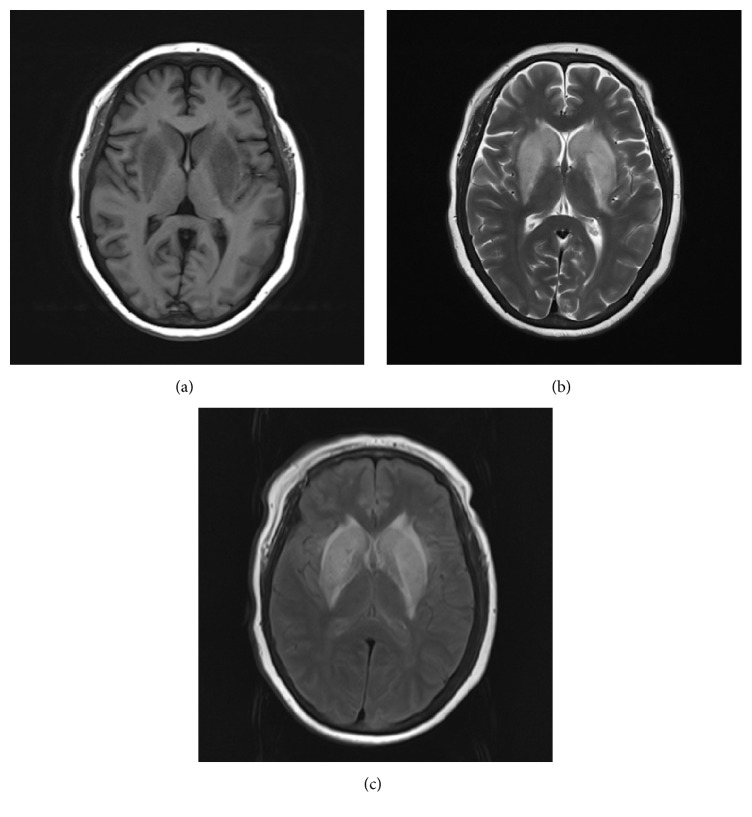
(a, b) The lesions extended in both T1WI and T2WI after 3 weeks. (c) FLAIR images showed demyelination around bilateral ventricles and lacunar infarctions.
